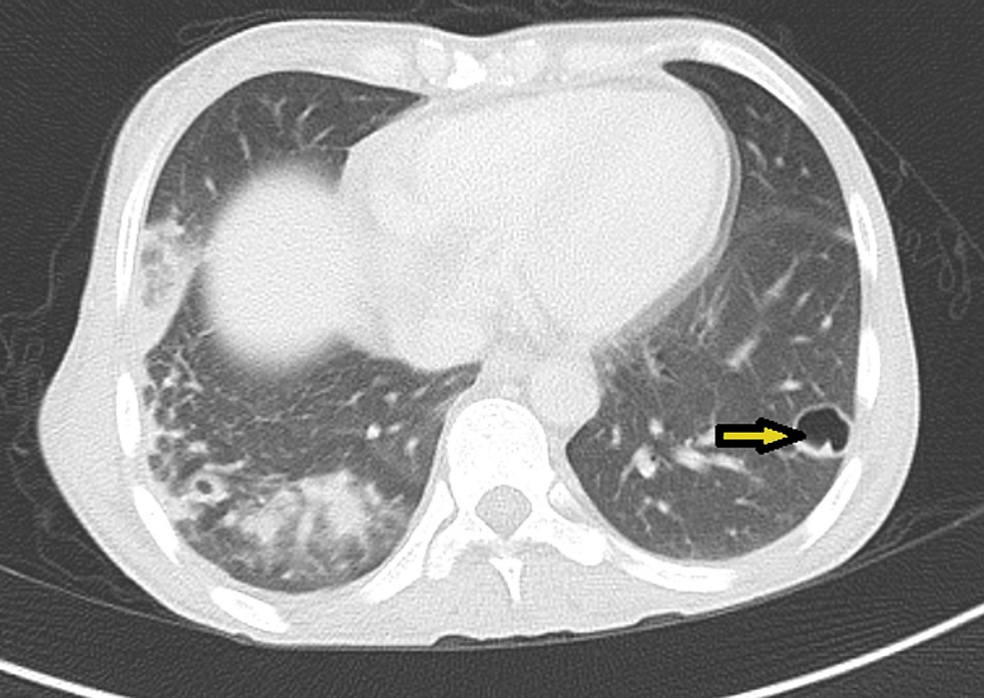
Thoracic CT scan of an axial slice showing pulmonary septic embolism.
